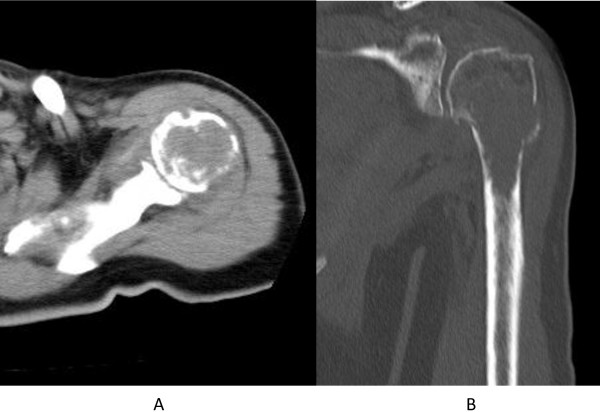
Computed tomography scan shows cortical destruction of the proximal humerus and a pathological fracture. (A) Transverse view. (B) Coronal view.
